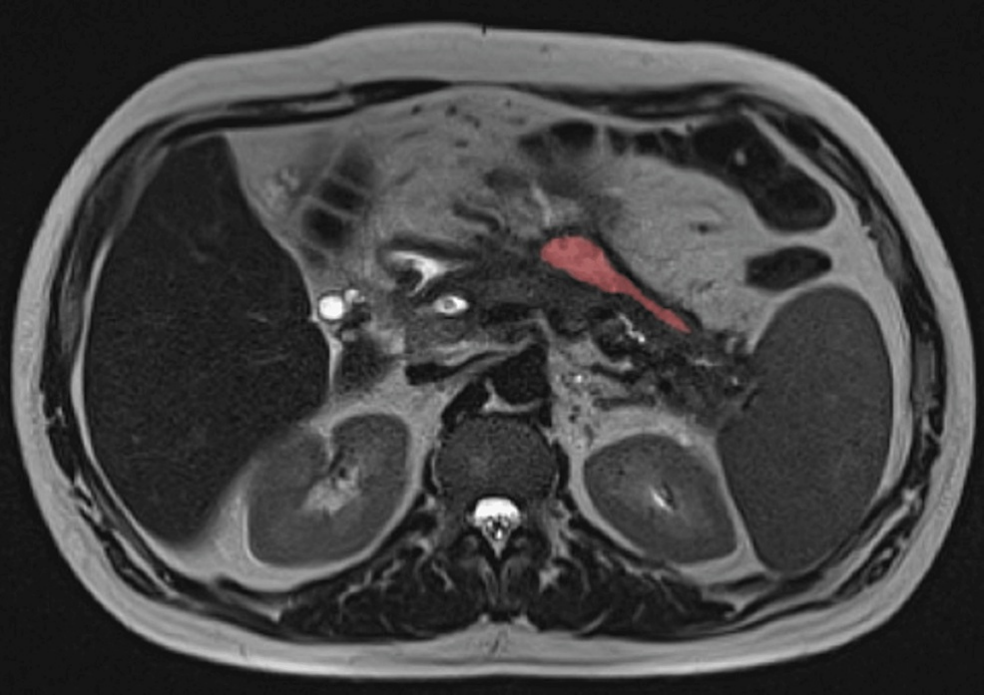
Small residual collection. Abdominal magnetic resonance imaging showing small residual collection (red).
